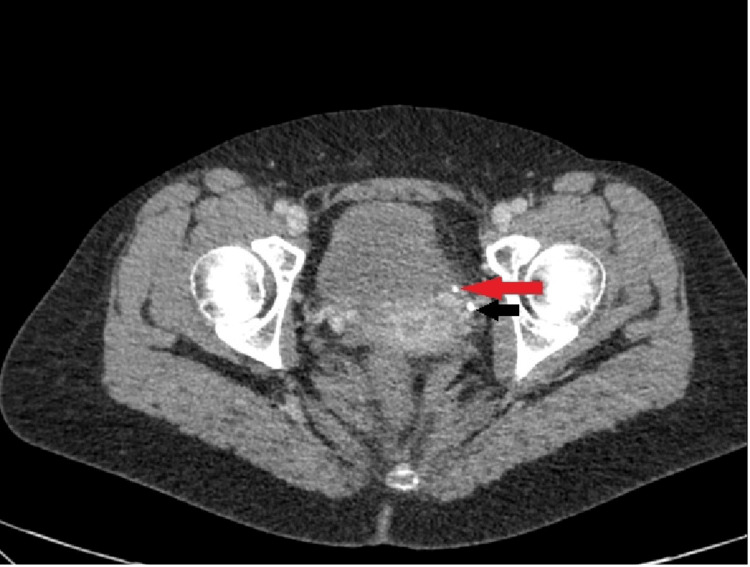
CT (axial view) of the pelvis showing the 3-mm obstructing stone at the left vesicoureteric junction  (red arrow), and associated inflammatory changes and a phlebolith (black arrow)
